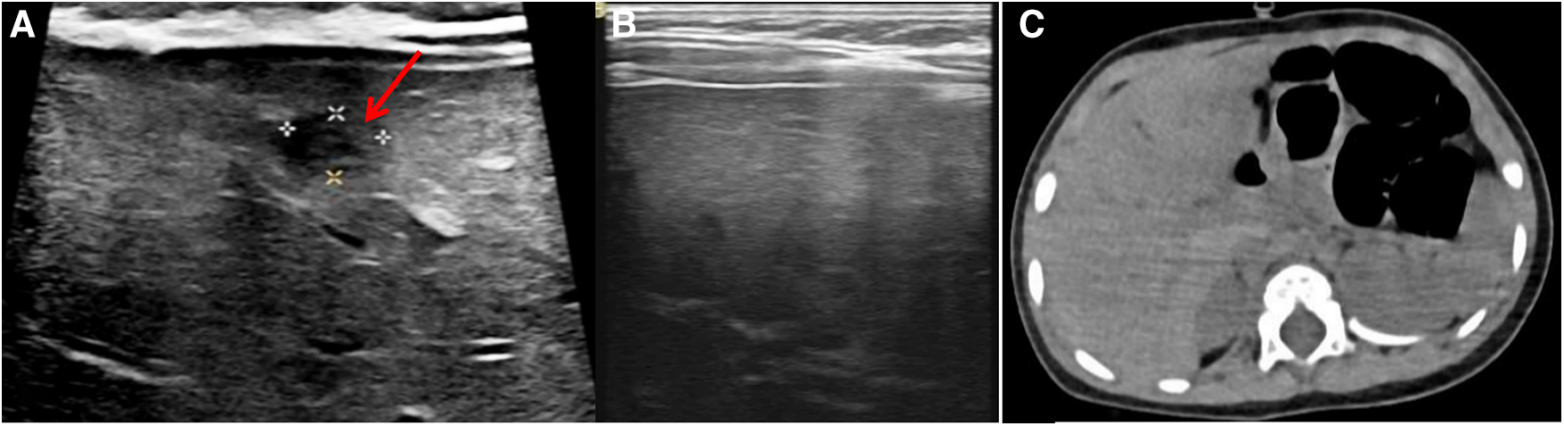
Post-discharge follow-up imaging results. (A) Abdominal ultrasound (one month after discharge): liver abscess had shrunk (Red arrow, 3.5 mm × 2.8 mm). (B) Abdominal ultrasound (three month after discharge): liver abscess had absorbed. (C) Abdominal CT scan (eight month after discharge): no liver parenchymal lesions.
